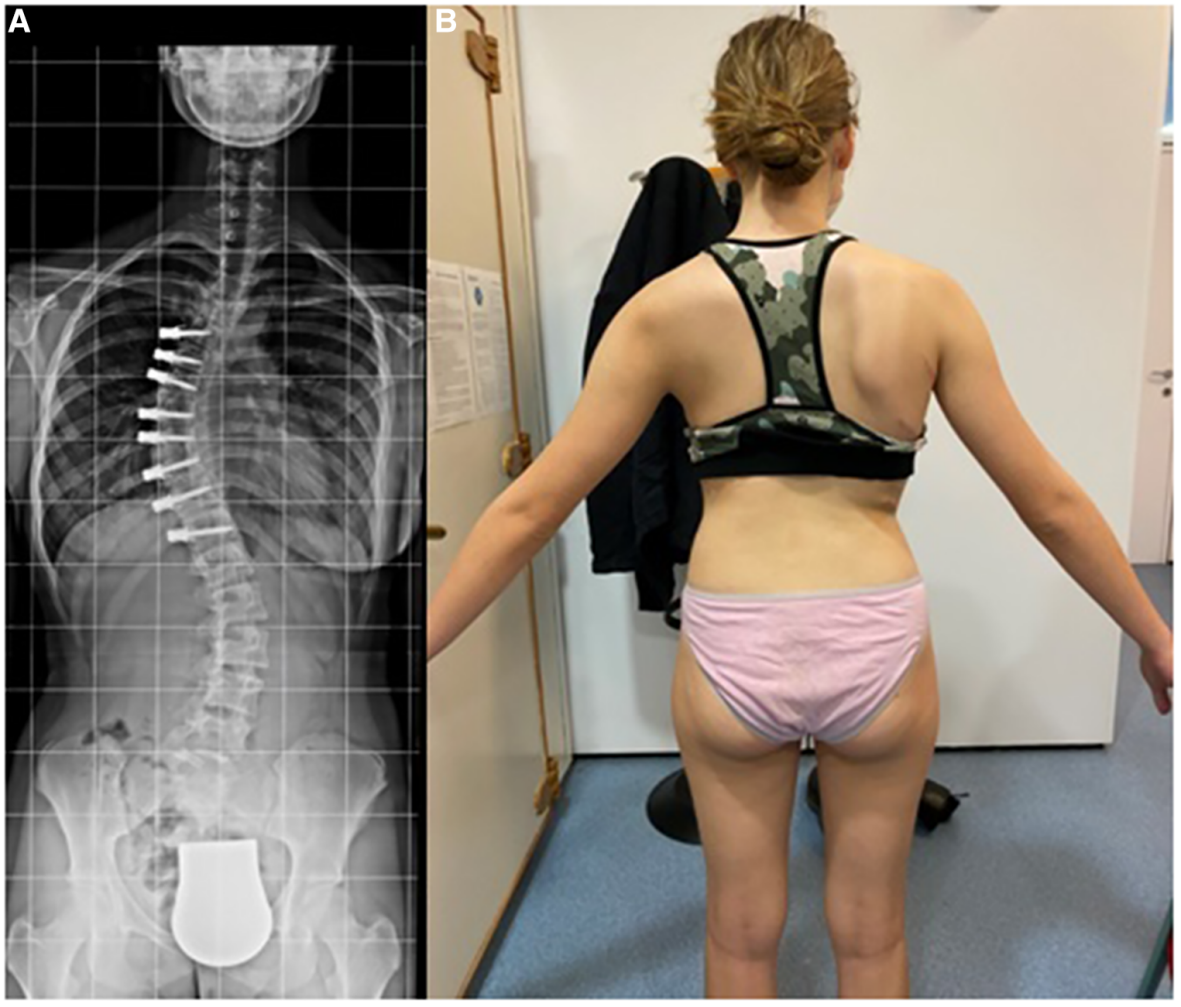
1-year post-tethering, patient is well compensated; (A) radiographic compensation, (B) apparent compensation.
